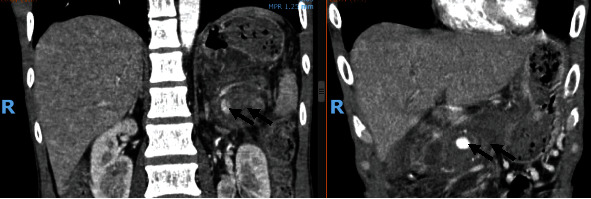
Coronal contrast-enhanced computed tomographic images reveal two pseudoaneurysms (black arrows: pseudoaneurysm filling with contrast).
